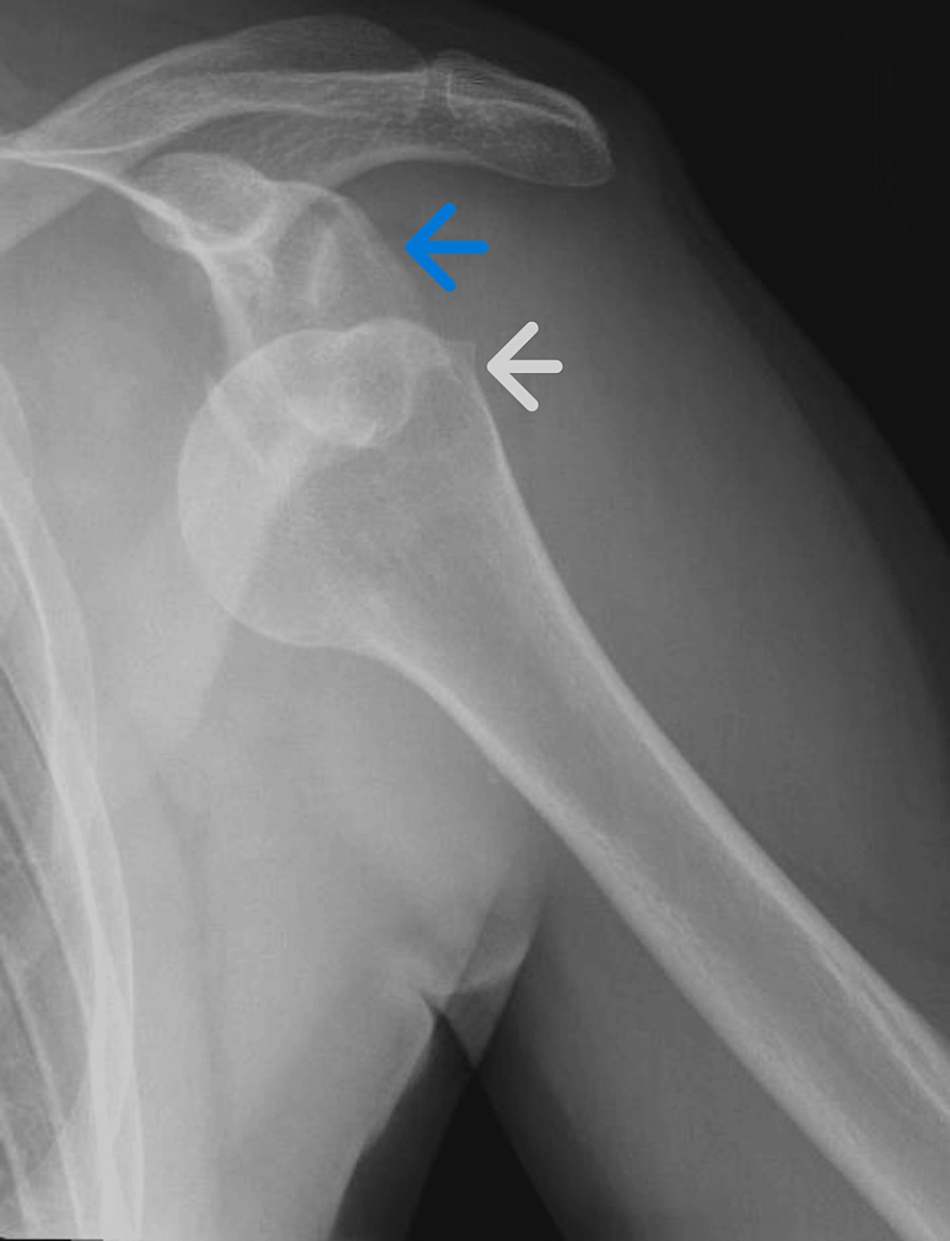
X-ray prior to reduction showing anterior shoulder dislocation Glenoid fossa (blue arrow); humeral head (white arrow)
